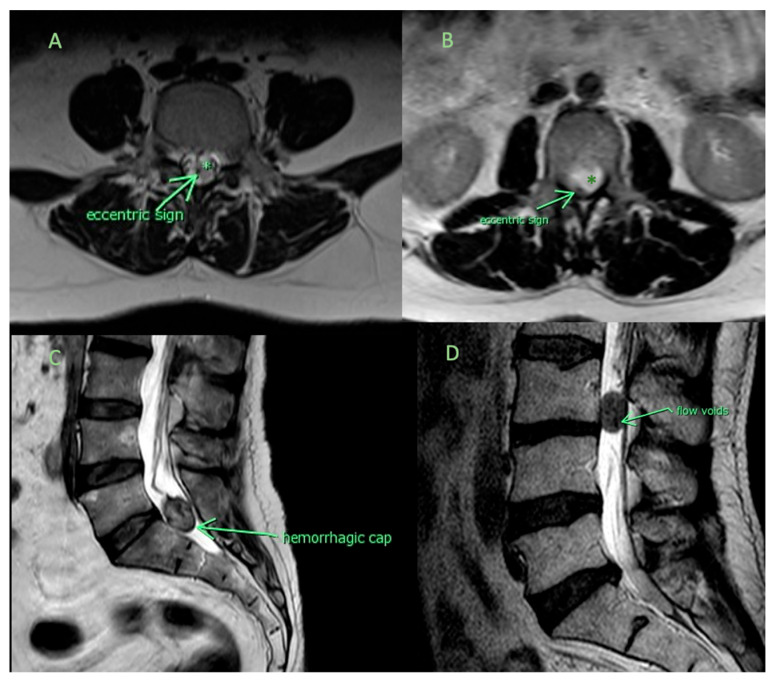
Representative magnetic resonance imaging findings of CENETs. Axial T2-weighted images (A,B) demonstrate the “eccentric vessel sign” (arrows), characterized by a serpiginous vascular structure located eccentrically within the tumor. Sagittal T2-weighted image (C) shows a lesion with a “hemorrhagic cap sign” (arrow), representing a hypointense rim due to hemosiderin deposition. Sagittal T2-weighted image (D) demonstrates prominent intralesional and peritumoral flow voids (arrowheads), consistent with the hypervascular nature of these tumors.
